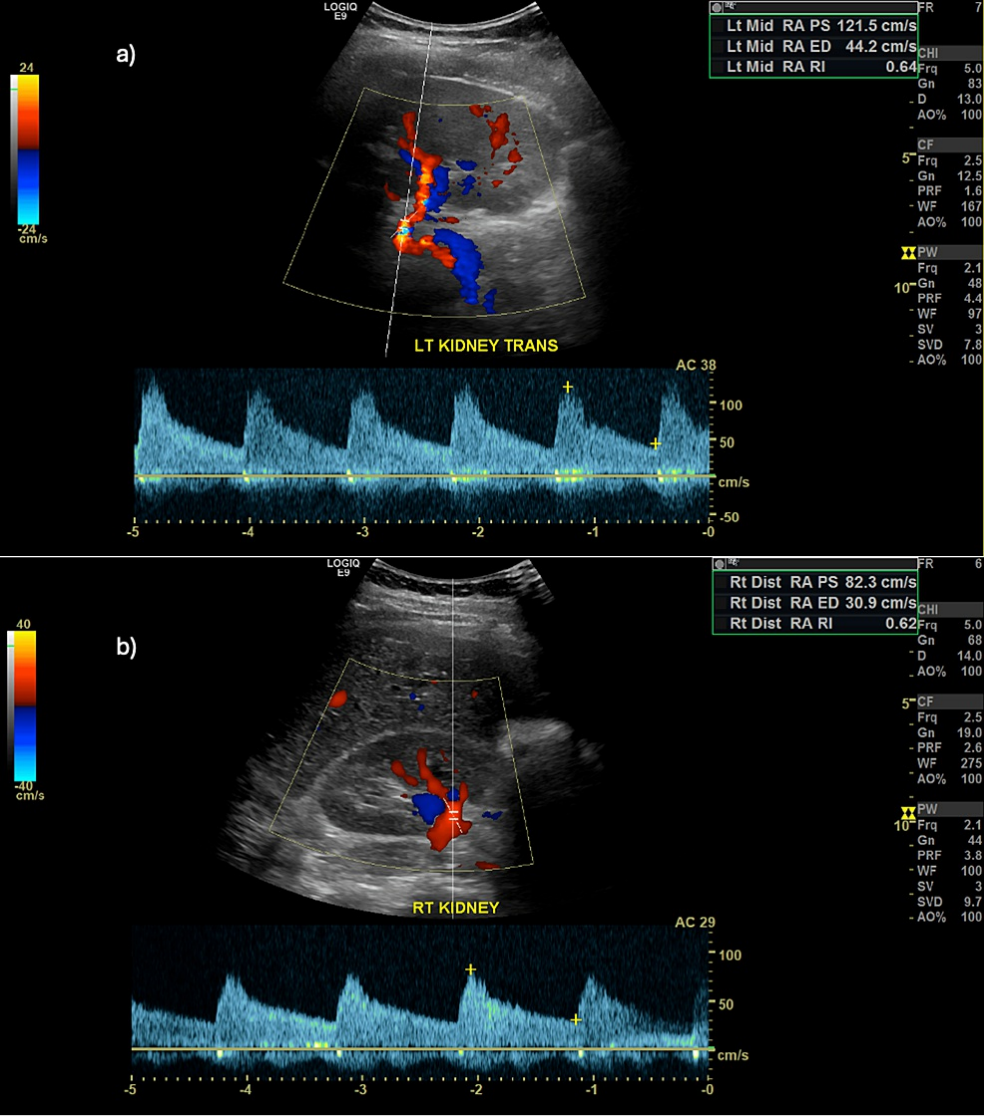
Renal duplex ultrasound a) Good arterial flow in the left mid-renal artery
